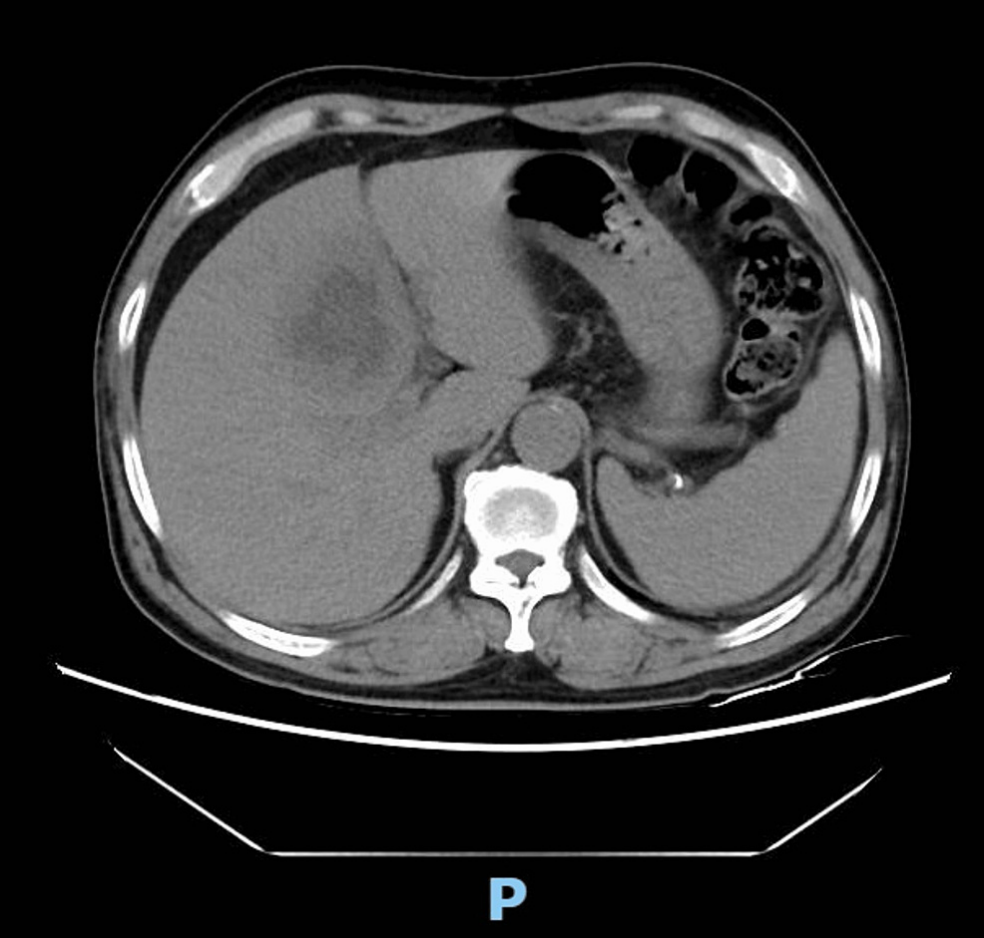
Abdominal computed tomography scan showing a hypodense lesion with irregular margins in segment IV (arrow).
